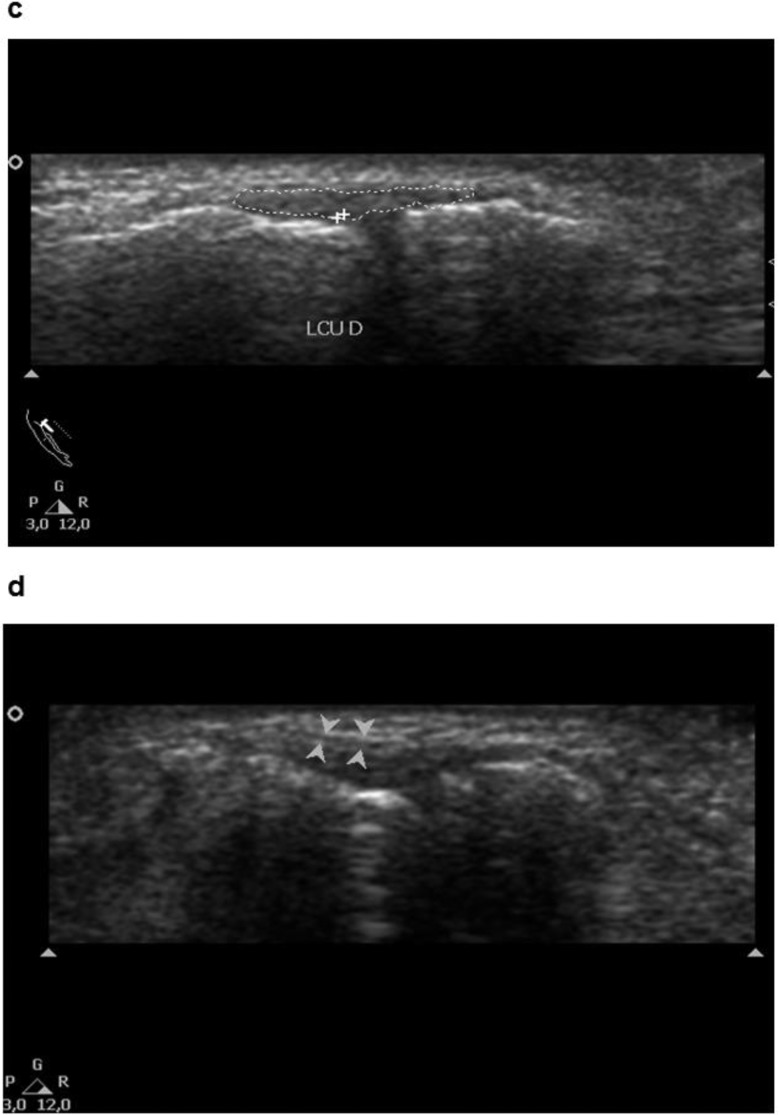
Ultrasonographic images showing the perimeter area of the ligament (c) and aponeurosis of the adductor pollicis muscle (d).
